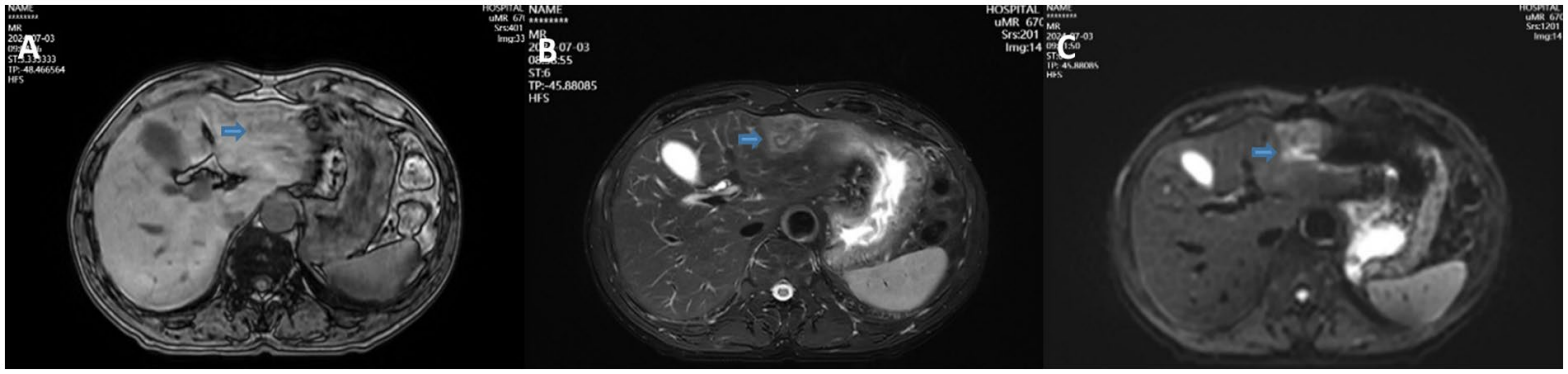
T1WI (A), T2WI (B), and DWI (C) showing a solid low-density mass in the left lateral lobe of the liver.
